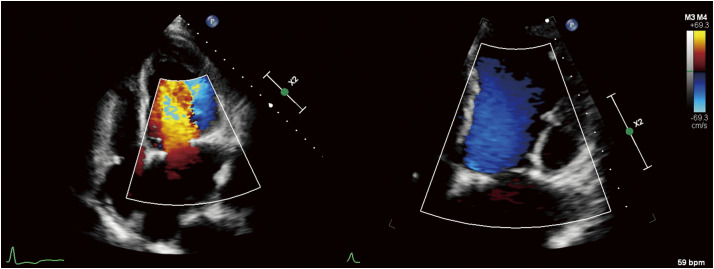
Postoperative echocardiography findings. Postoperative echocardiography revealed no residual MR, an MV area of 2.6 cm^2^, a transmitral pressure gradient of 10/2 mmHg, and an LV EF of 40%. EF: ejection fraction; LV: left ventricle; MR: mitral regurgitation; MV: mitral valve
